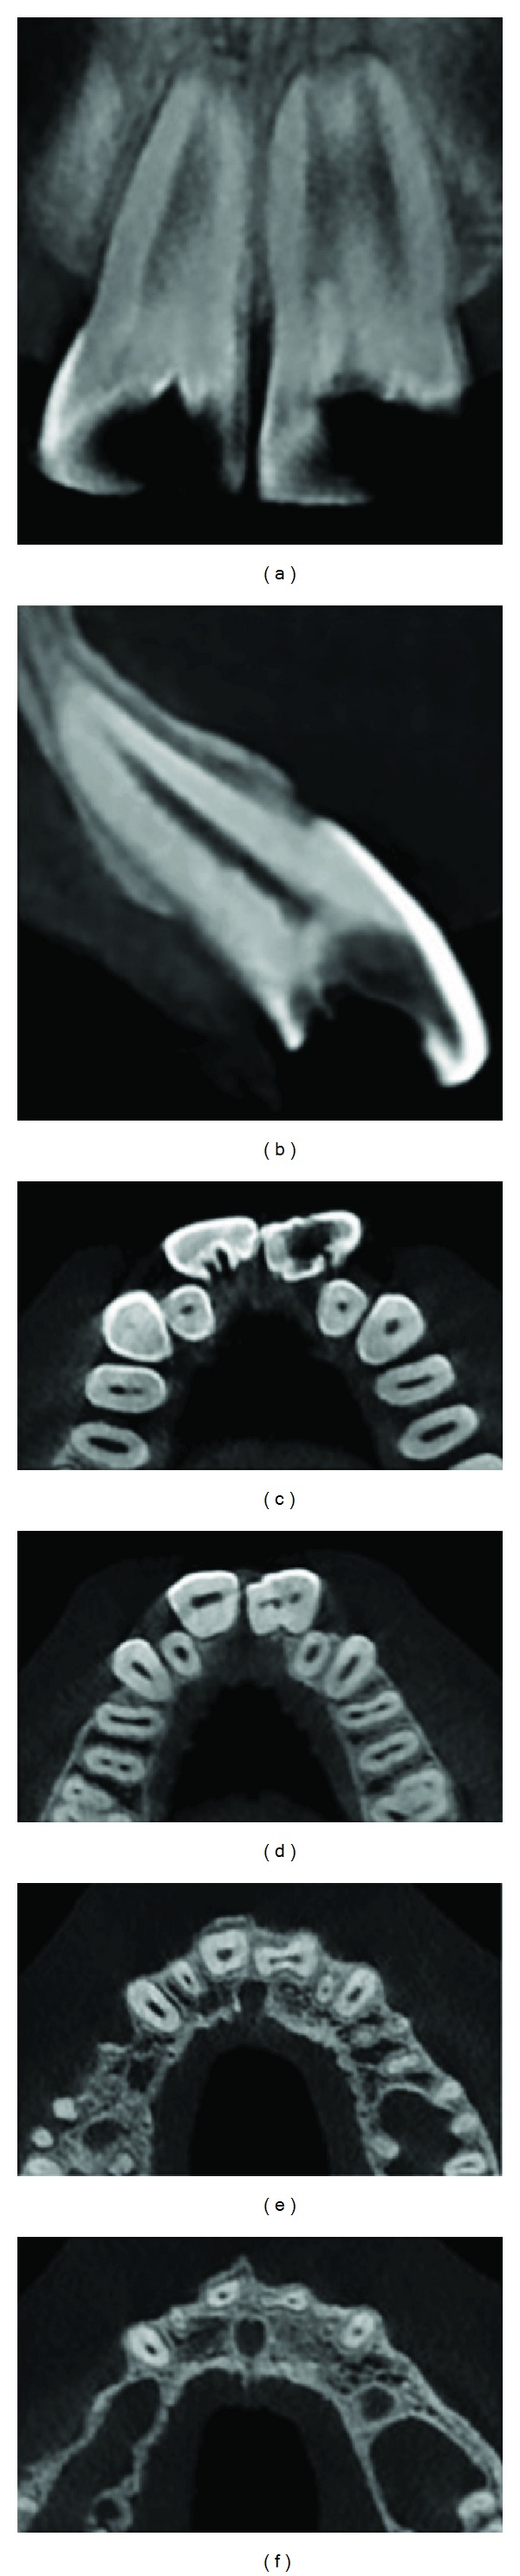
Different cone beam computed tomography (CBCT) sections of geminated incisors.
